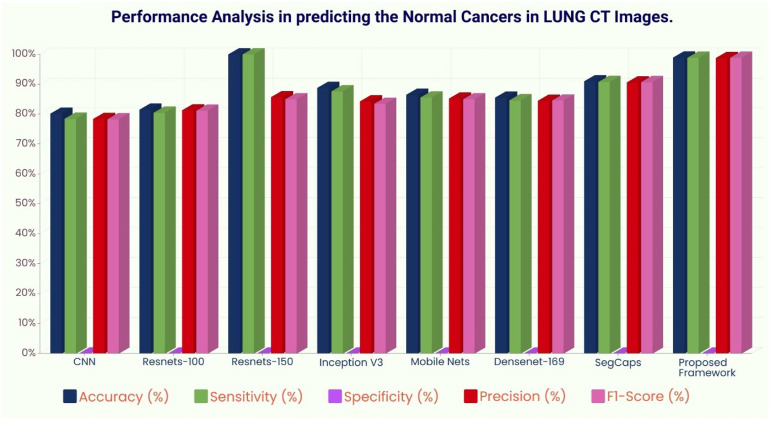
Performance analysis in predicting normal tissue in lung CT images.
